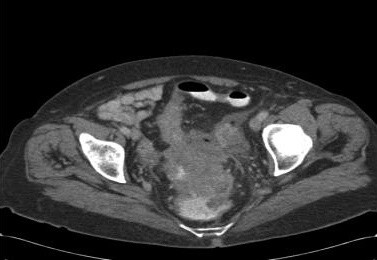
CT scan demonstrating a rectosigmoid mass with local perforation.
